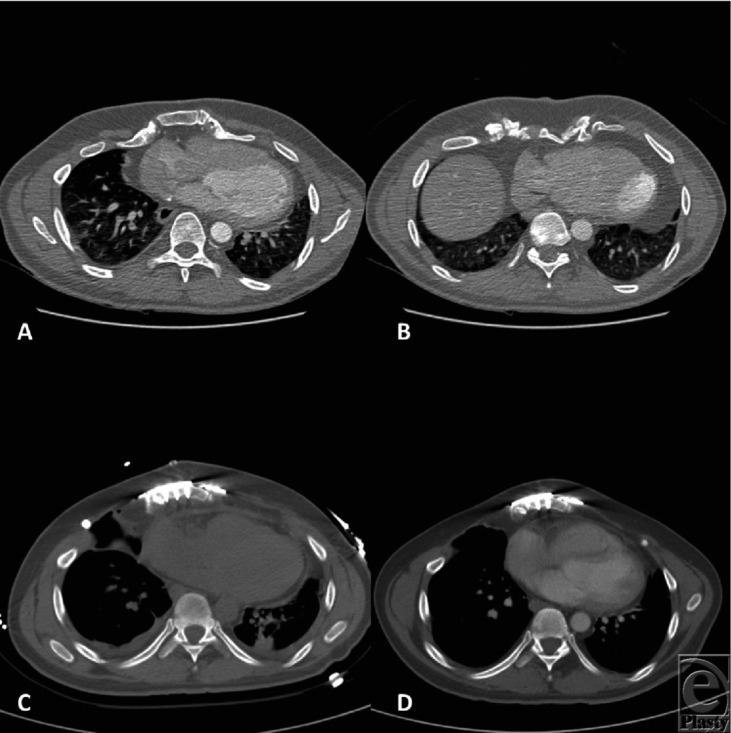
(*a* and *b*) Preoperation computed tomography showing concavity. (*c*) Postoperative at 5 days, showing relief of RV pinch. (*d*) At 4 months, there continues to be relief of RV pinch. There is also remodeling of heart, allowing for greater left lung capacity. RV indicates right ventricle.
